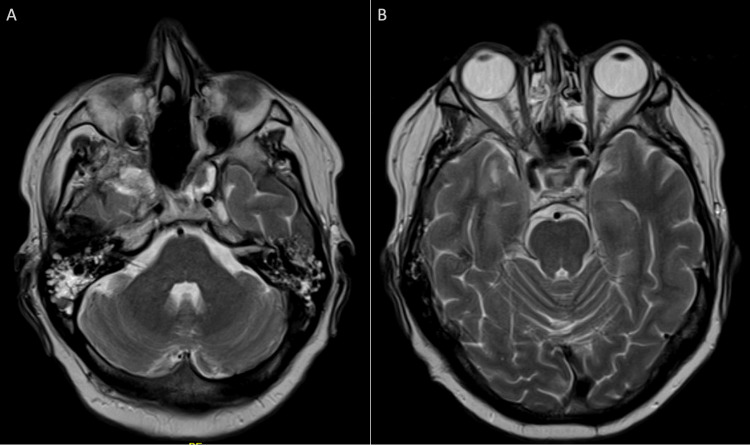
MRI of the brain with contrast (four weeks post-radiothrapy completion). Axial T2-weighted MRI images (A) at the level of the posterior cranial fossa and (B) at the level of sella turcica. MRI of the brain with contrast performed four weeks after completion of radiotherapy shows a marked reduction in tumour size following treatment.
